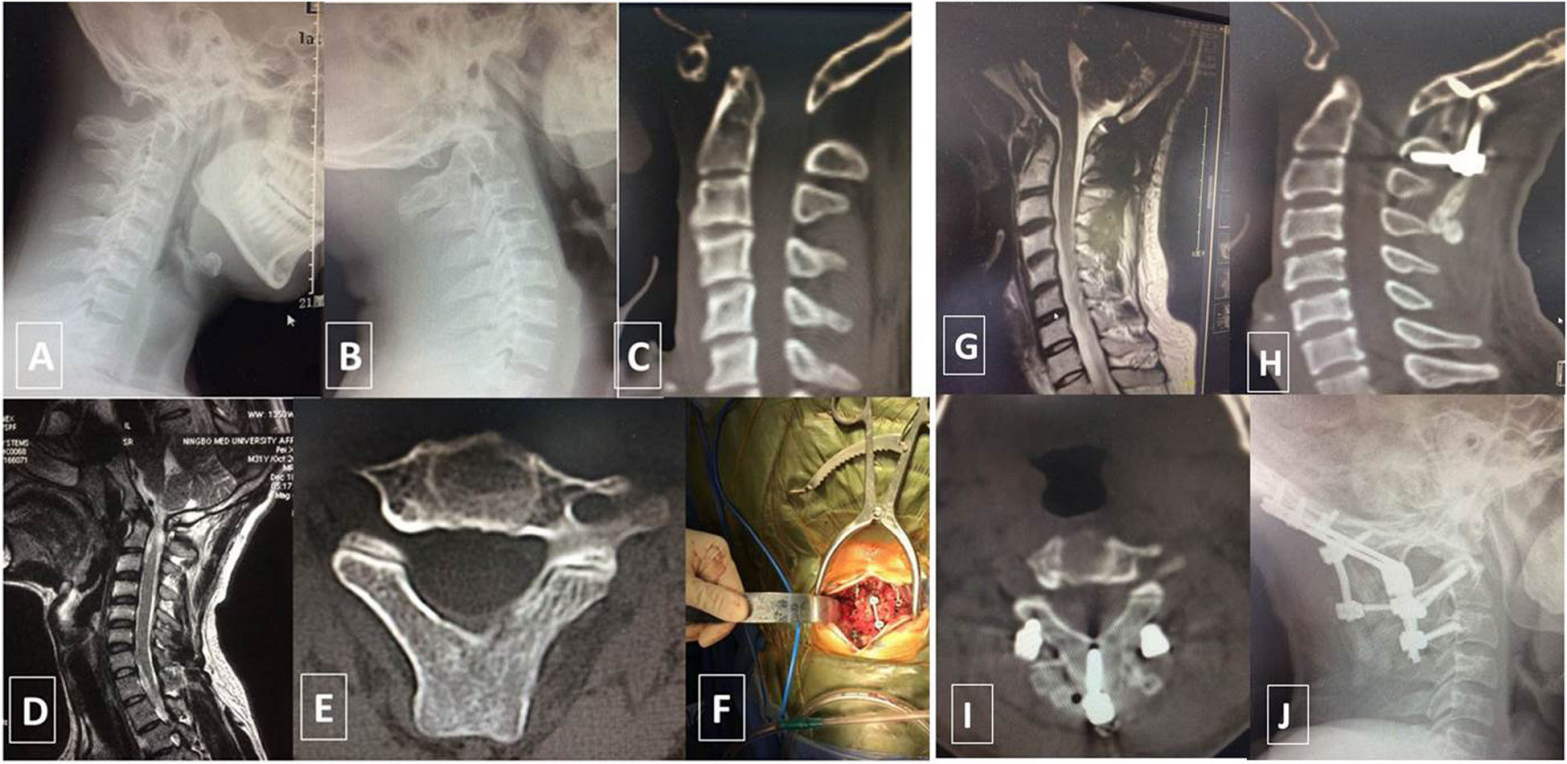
A 35-year-old man with occipitocervical deformity was treated with posterior occipitocervical fixation and fusion. a–c Occipital screws, C2 bilateral pedicle screws, a C2 spinous process screw, and C3 bilateral lateral mass screws were placed. Preoperative dynamic radiography and computed tomography (CT) reconstruction images show the occipitocervical deformity and instability. d Preoperative magnetic resonance imaging (MRI) shows the occipitocervical deformity and spinal cord compression. e Preoperative CT shows that the C2 spinous process was relatively broad and thick. f Intraoperative image shows the good position of the screws. g Postoperative MRI shows full decompression of the occipitocervical region. h, i Postoperative CT shows that the C2 spinous process screw was placed correctly, achieving successful occipitocervical fixation. j Follow-up lateral-view radiography shows that a good occipitocervical sequence was achieved, with no loosening of the internal fixation
